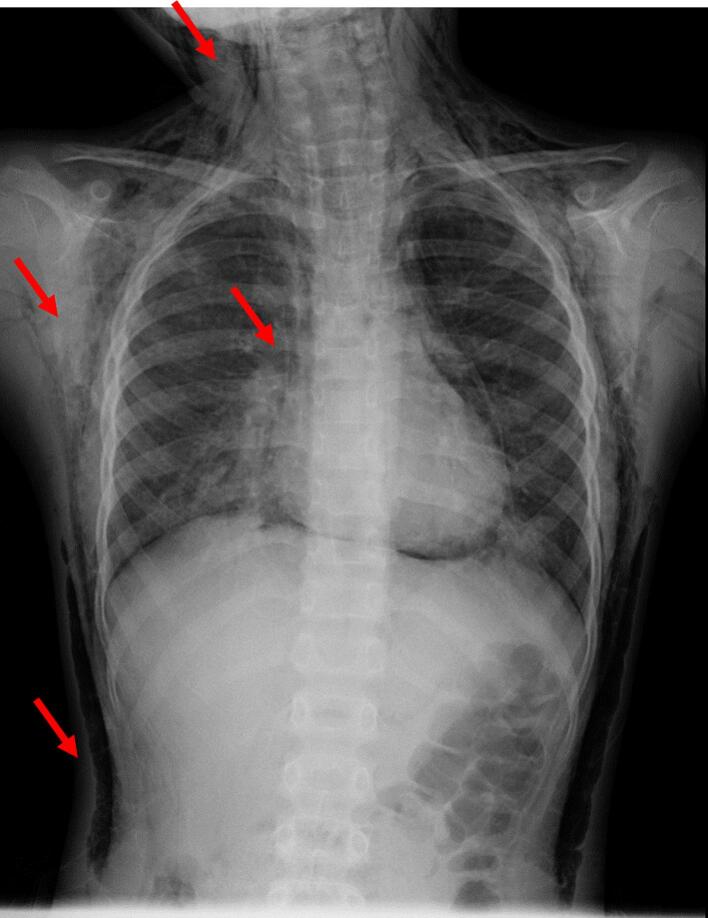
A frontal chest radiograph showing pneumomediastinum (red arrows in the middle) and subcutaneous emphysemas (red arrows in the upper and lower chest).
